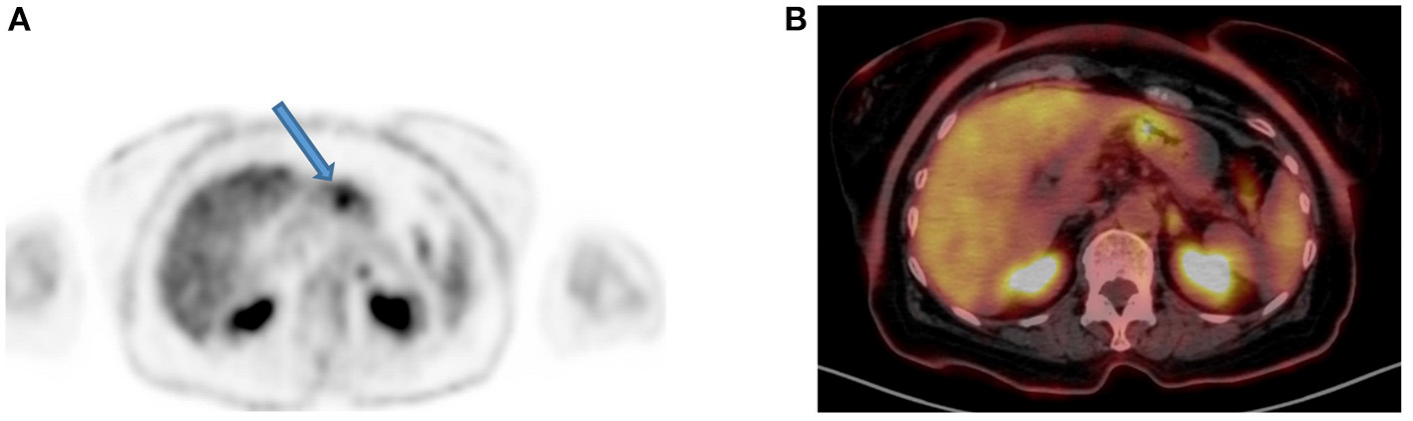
Focal gastric uptake. A 76-year-old female with newly diagnosed poorly differentiated gastric adenocarcinoma. (A) A focus FDG PET seen in Axial image (arrow) and (B) confirmed in the combined PET/CT. Focal uptake in gastric wall is typical for carcinoma and there was gastric outlet obstruction seen.
